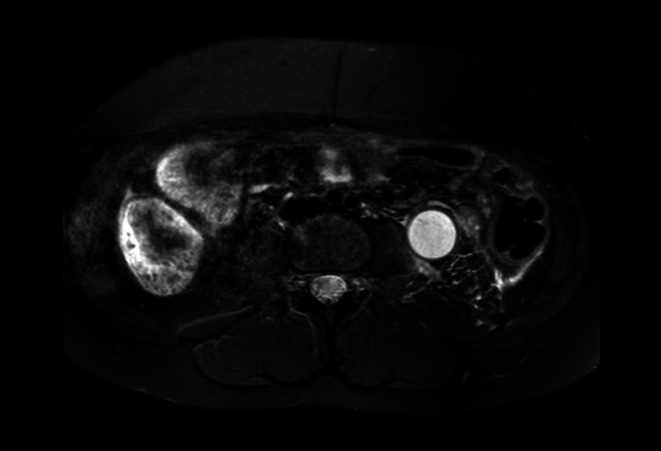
Multiparametric pelvic MRI, axial T2W SPIR HR sequence showing ectatic left megaureter with diffusely overfluid contents and concentrically thickened and hyperintense walls.
